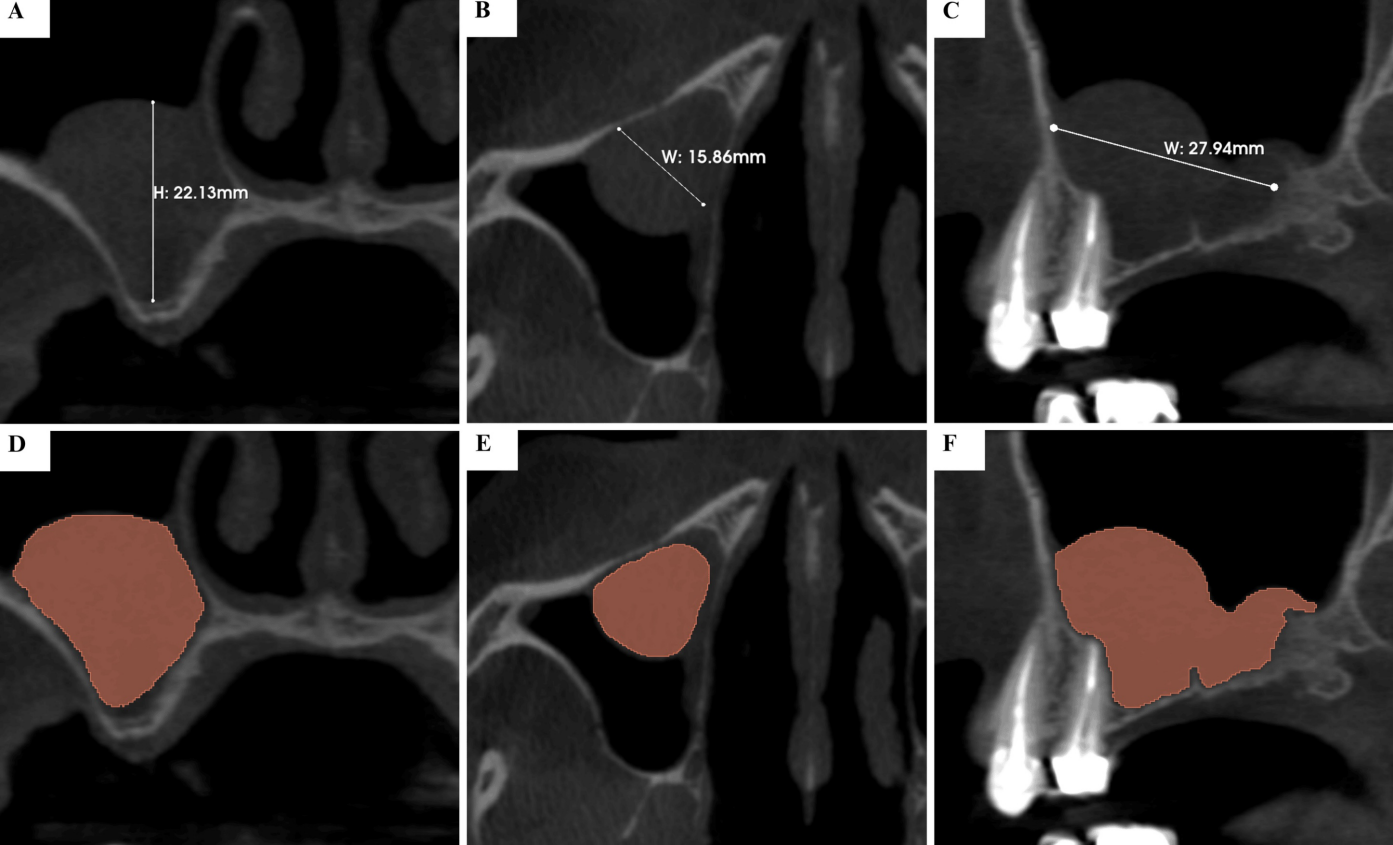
Preoperative Cone-Beam Computed Tomography (CBCT) of the Right Maxillary Sinus Showing a Mucous Retention Cyst Preoperative CBCT at baseline (T0) showing a probable mucous retention cyst, well-defined and dome-shaped, in the right maxillary sinus. The lesion measured 22.13 mm in height (A), 15.86 mm in bucco-palatal width (B), and 27.94 mm in mesio-distal width (C), occupying a substantial portion of the sinus and closely related to the planned implant site in the edentulous posterior maxilla. The brown region of interest (ROI) highlighted in panels (D)-(F) corresponds to the area of the probable mucous retention cyst within the right maxillary sinus.
